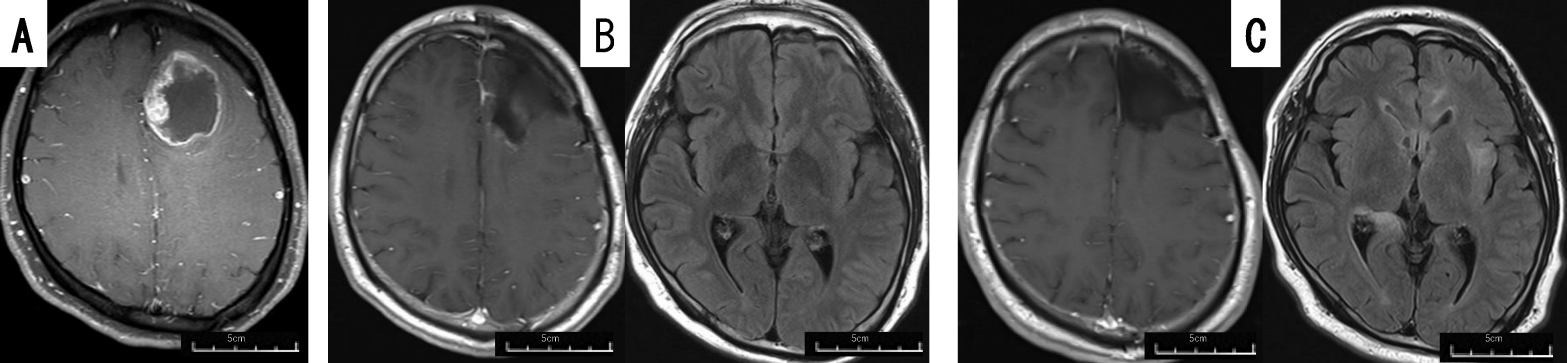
Patient 11 (30-35 year-old man): GBM, *IDH*-wild, demonstrating dissemination 20 months after PDT+TTF. Preoperative gadolinium-enhanced brain MRI (A) of patient 11, displaying a lesion in the left frontal lobe. Postoperative gadolinium-enhanced (left images) and FLAIR (right images) brain MRI taken 2 months (B) and 20 months (C) after the operation. The brain FLAIR MRI taken 20 months after the operation displayed dissemination around the lateral ventricle and left insular lobe.
